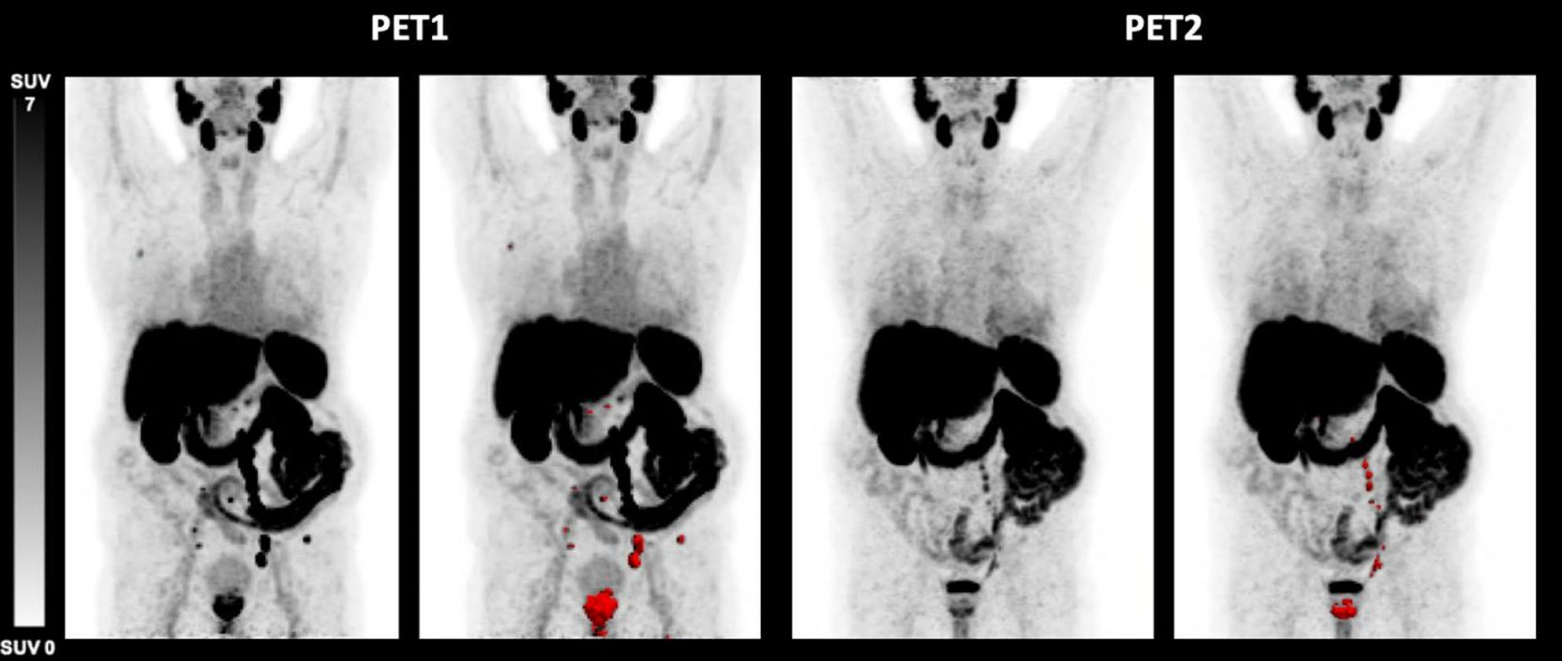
[^18^F]PSMA-1007 PET/CT derived maximum intensity projection (MIP) of a 79-year-old patient with metastatic prostate cancer before (PET1) and after (PET2) initiation of apalutamide therapy (time difference 5.00 months). TTV (− 34.7%) and SUV_max_ (− 58.6%) decreased, SUV_mean_ (+ 19.6%) increased, PSA level decreased from 25.00 ng/mL to 0.03 ng/mL. This patient was rated as PR according to RECIP 1.0
